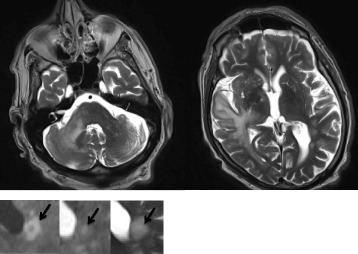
Brain MRI on day 38 of admission in a male with underlying PCV who developed PML. Axial T2-weighted sections display increased number of widespread and confluent supra- and infratentorial lesions. There were several “ring-shaped” lesions with hyperintensive periphery on DWI, increased central signal and isointense periphery on ADC. On T2-weighted images the lesions were globally hyperintense
